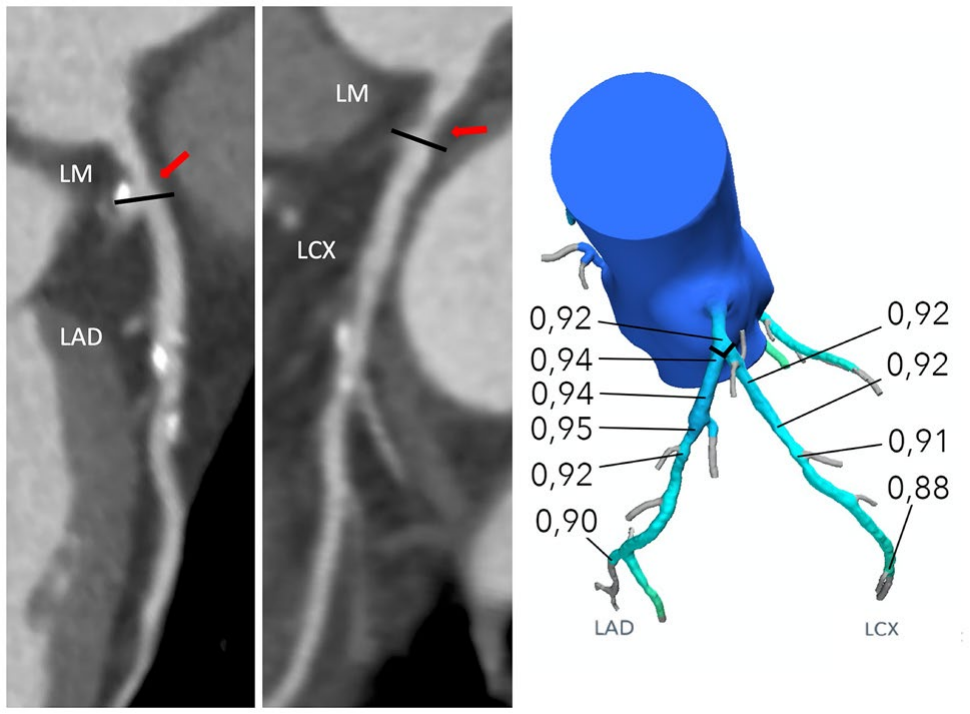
Pressure recovery phenomenon. Typically, pressure will reach a minimum in the throat of stenosis with slight pressure recovery 0.5–1 cm distal to the stenosis because of the increase in the cross-sectional area of the vessel and then decrease further downstream the vessel due to the continuous decrease in the cross-sectional area of the vessel and possibly the presence of flow limiting artery disease in more distal segments. However, FFR_CT_ values may transiently rise also in segments located more distal to stenosis. In this case, the step-up in FFR_CT_ from 0.92 distally in the left main (LM) to 0.95 in the mid left anterior descending artery (LAD) is caused by the presence of post-stenotic vessel dilatation resulting in reduced flow velocity and pressure recovery. We defined significant pressure recovery as an increase in FFR_CT_ ≥ 0.03 when moving from the lesion-specific FFR_CT_ "reading point" (typically 1–2 cm distal to the lower border of the stenosis) to more distal located segments. Coronary CT angiography curved multiplanar reconstructions. Right: Three-dimensional FFR_CT_ model. Red arrow indicates the location of LMCAD. *LCX* left circumflex artery
